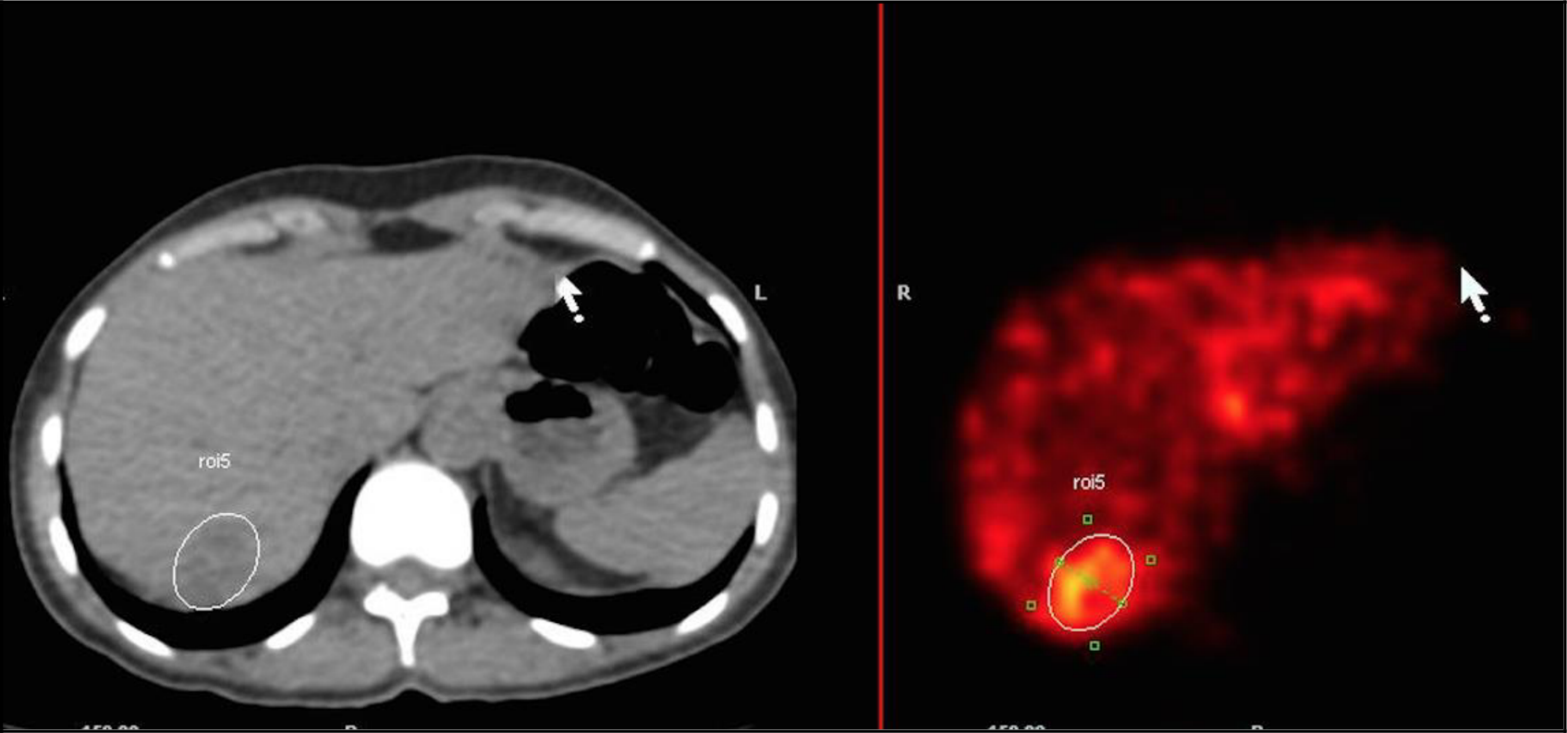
Gamma counts measurement in SPECT/CT after establishing ROI on CT scan with the longest tumor diameter.
